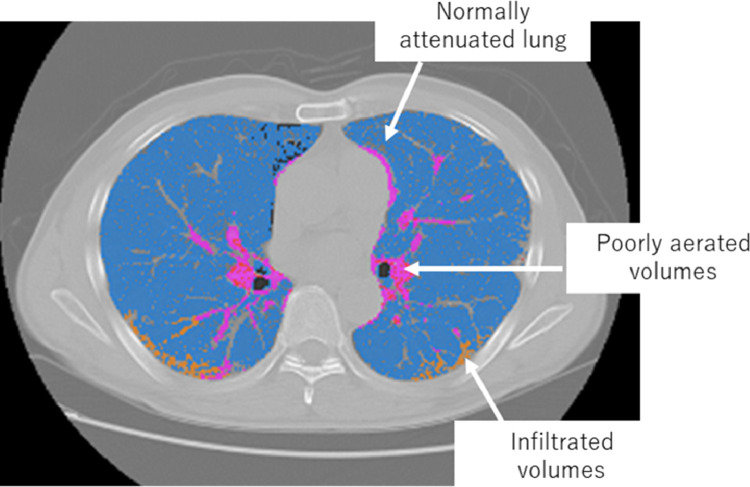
Chest computed tomography (CT) image for IPF patient. The CT was segmented by the threshold CT values. The blue shows normally attenuated patters, the pink shows poorly aerated pattern, and the orange shows infiltrated pattern.
